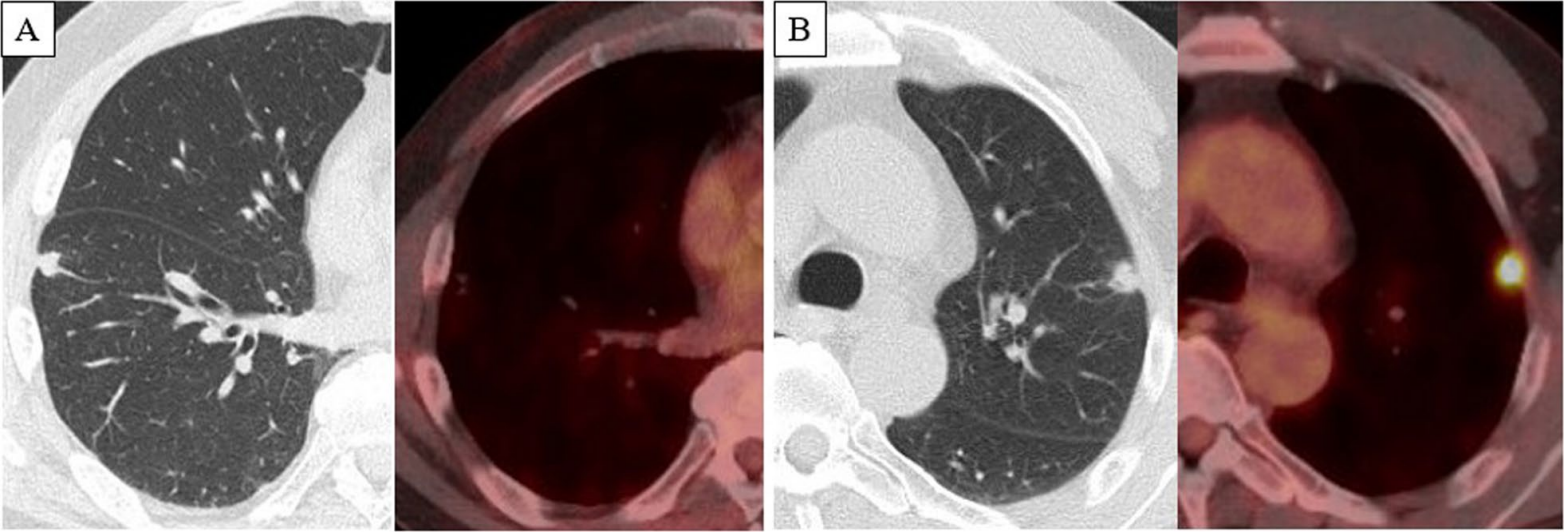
Follow-up CT and FDG-PET/CT at 2 years after treatment. FDG-PET/CT showed a poor FDG uptake in the right S8 10-mm nodule (A) but showed FDG uptake (SUVmax 10.7) in the left S3 15-mm nodule (B)
